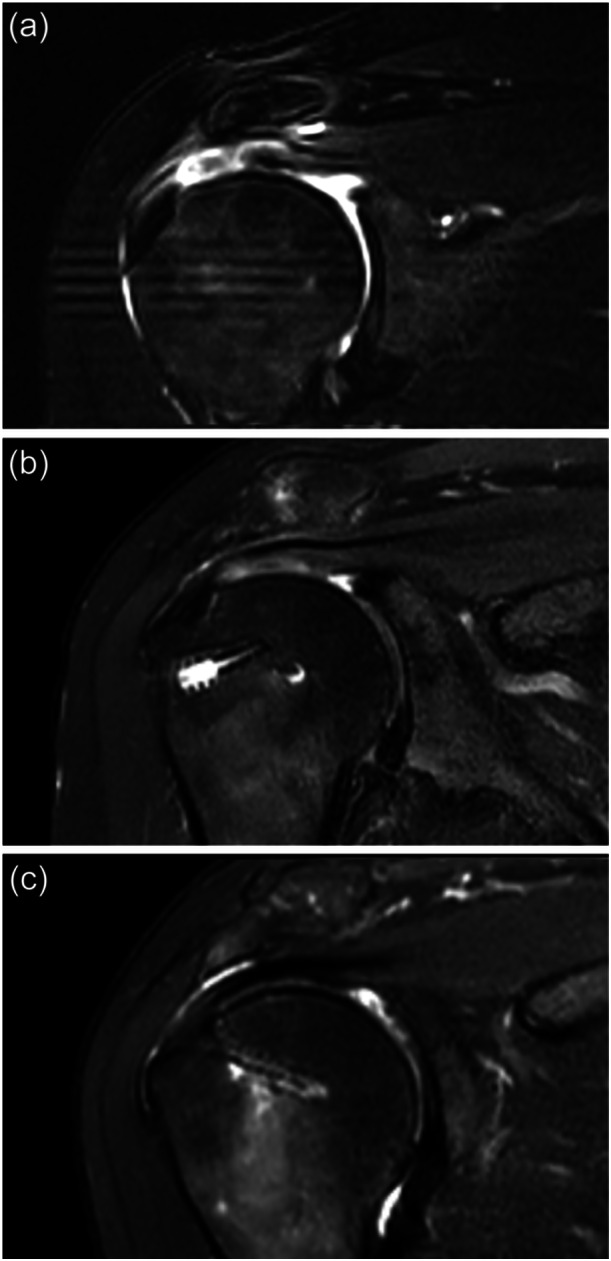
(a) Magnetic resonance image (MRI; coronal view T2) that shows a full‐thicnkess supraspinatus tear. (b) MRI image of the same patient 1 year after transosseous repair followed by Regeneten® implantation over the tear, showing healing of the tear with mild degenerative changes at the repair site. (c) MRI image of the same patient 2 years showing a healed tendon.
